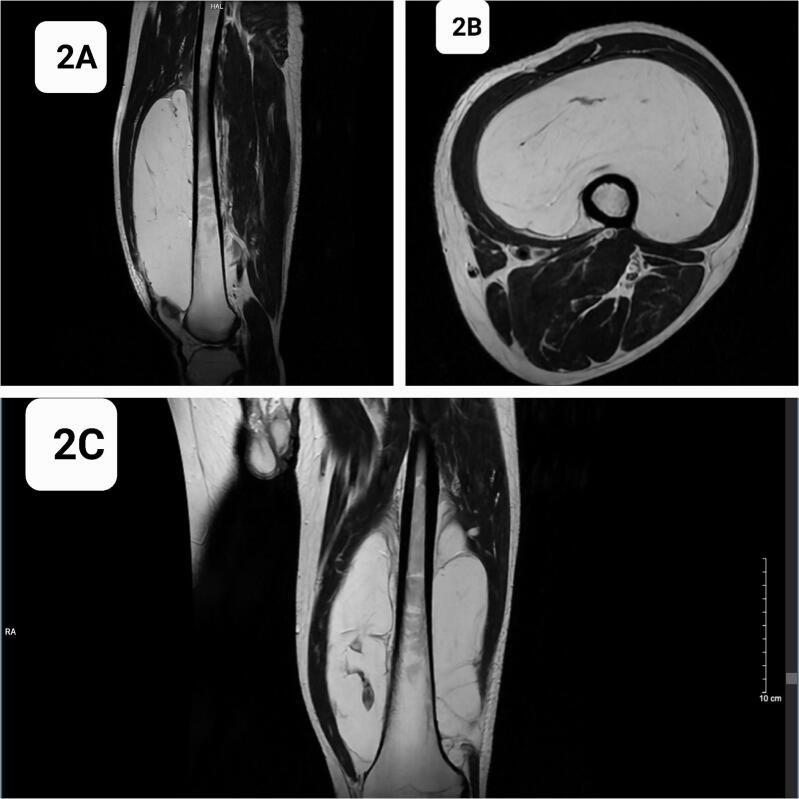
Giant lipomatous tumor around left femur on T2-weighted sequences MRI.
